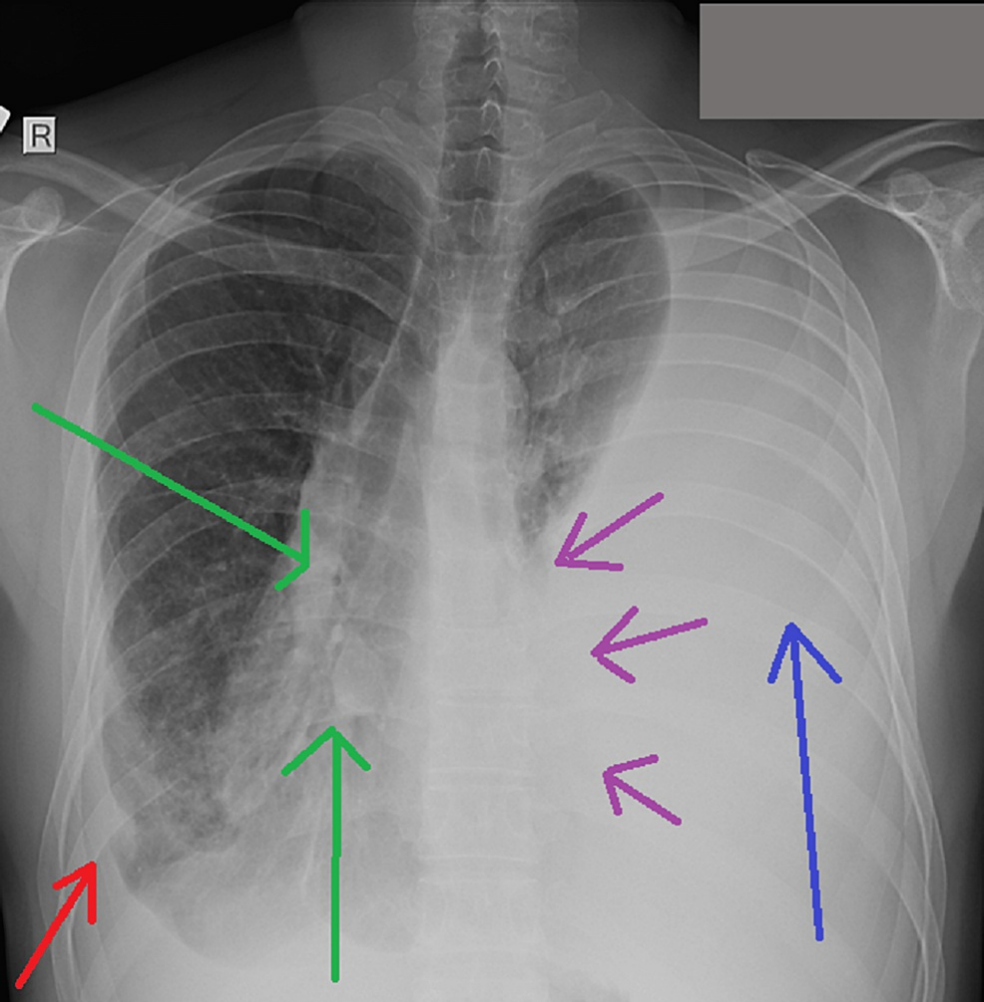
Admission chest X-ray showed a thickened pleura (red arrow) on the right side accompanied by a massive pleural effusion (blue arrow) on the left side. The cardiac silhouette is right-shifted (green arrows) with a blurred left side (purple arrows).
